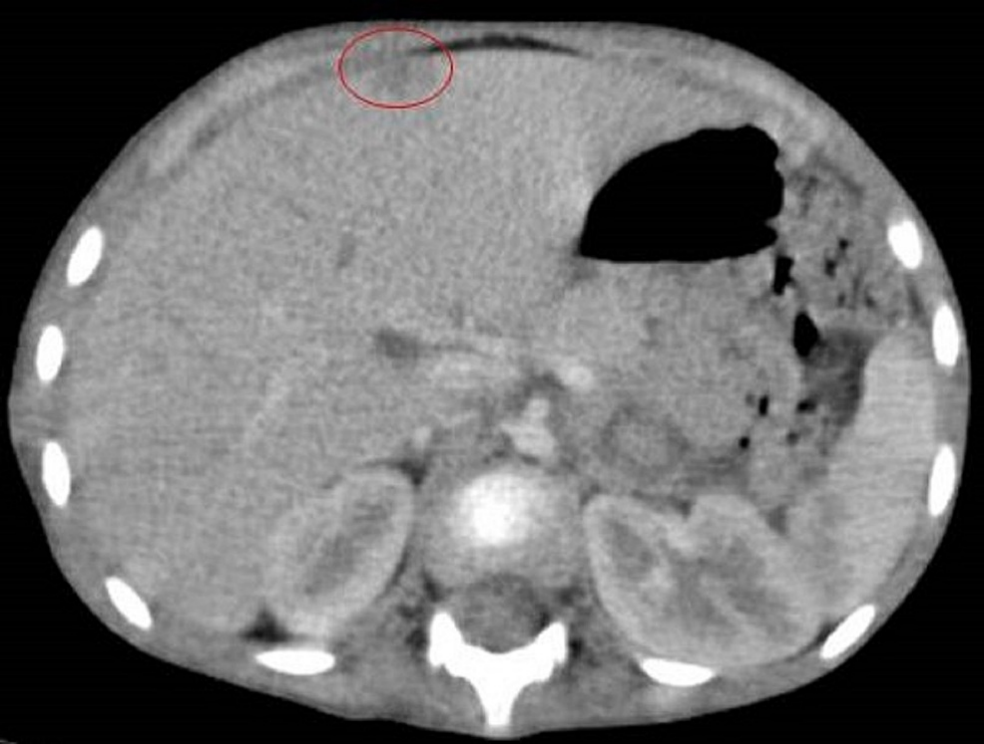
Thoracic CT scan CT scan showing a nodular hepatic lesion astride segments III and IV of secondary origin (circle).
